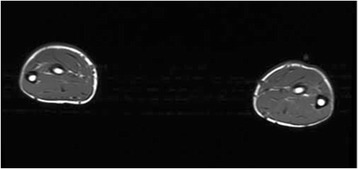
Bilateral forearm MRI pre-exercise
